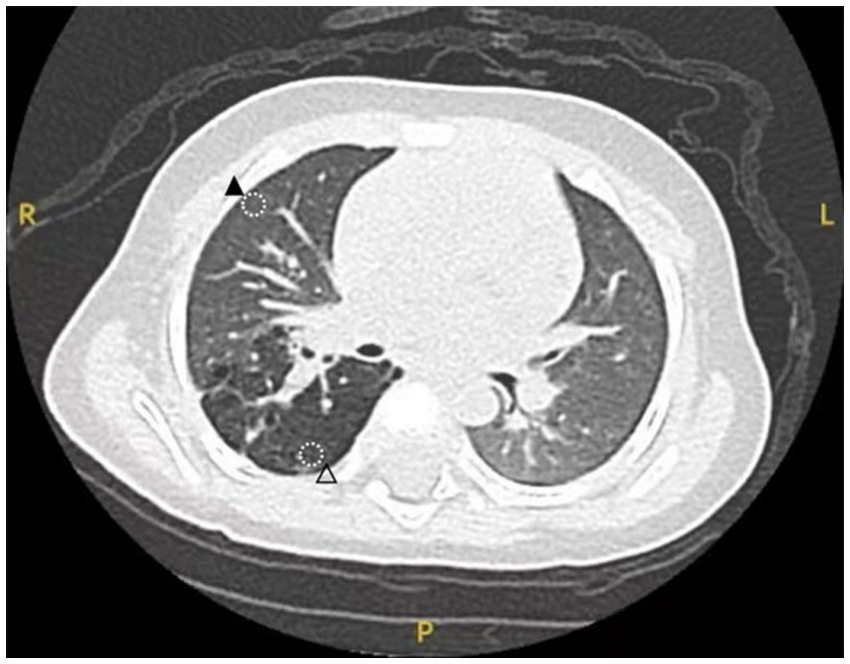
Preoperative CT image. ▲: Normal tissue sampling area. △: Lesion tissue sampling area.
